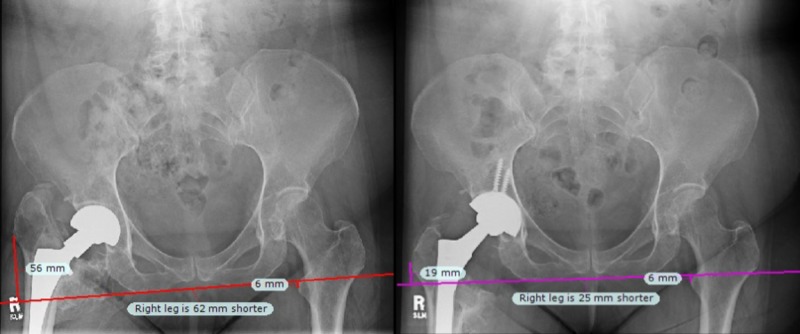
Radiographs for Case 2. Due to deformity at the operative lesser trochanter, the greater trochanter was used as a landmark for leg length calculations. Preoperatively (left), the LLD was estimated at 37 mm. Post-operatively (right), the operative leg was lengthened by 35 mm, which was confirmed by the navigation system and radiographs. LLD: Leg length discrepancy
